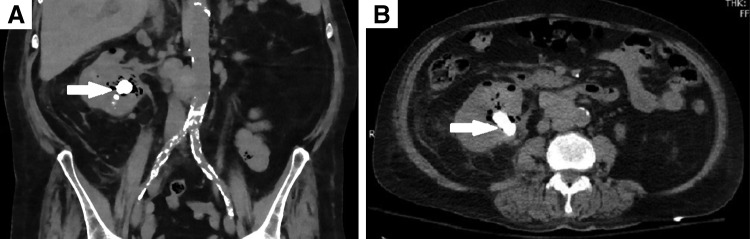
CT scan of the abdomen and pelvis without contrast in (A) coronal imaging and (B) axial imaging. The right kidney is enlarged containing a large amount of gas within the parenchyma and collecting system with a staghorn calculus in the renal pelvis measuring >3 cm (*white arrows*).
